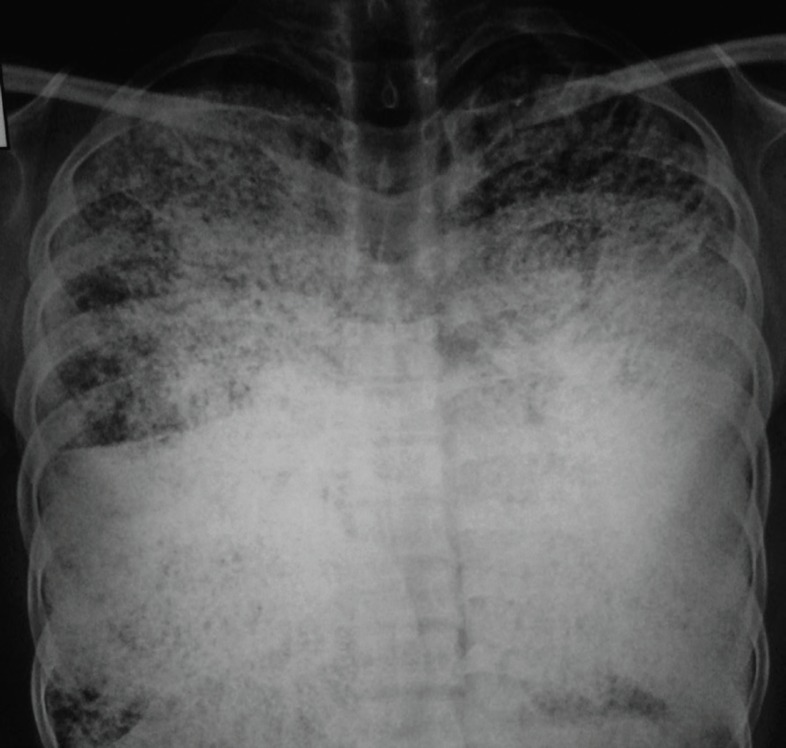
This chest radiograph (posteroanterior view) shows innumerable widespread, small, dense nodules, diffusely involving both lungs-predominantly in the basal regions - with obscuration of the mediastinal, cardiac, and diaphragmatic borders. A few fibrotic strands can be detected in bilateral lungs
